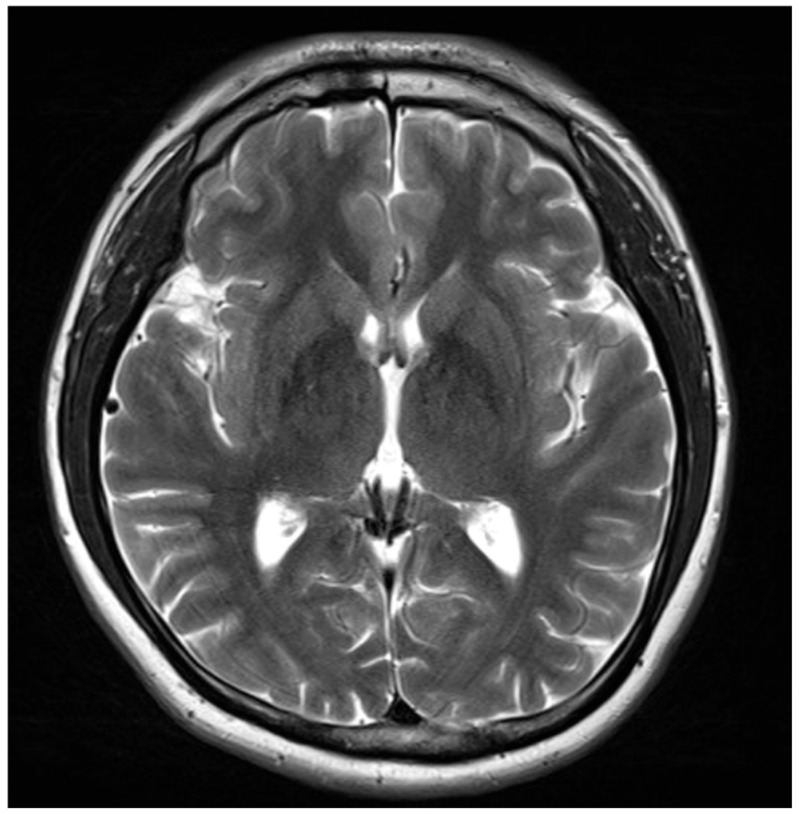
Magnetic resonance imaging of brain, which was normal.
